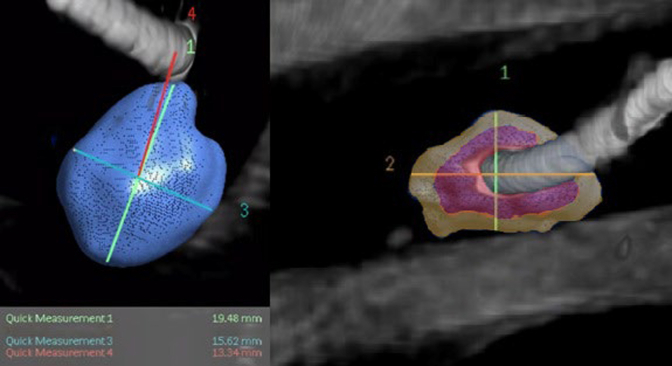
A, Quantitative assessment of tool distance from center of the target. Using this image obtained from cone beam CT scan, the center of the nodule is found (intersection between green and teal lines). A measurement is then obtained from the center of the target to the center of the catheter (red line) to determine the distance from the center; in this case, it is 13.34 mm (or 4.45× catheter diameter). The needle is not seen here because it has been deployed away from the viewer. All nodule measurements were obtained with the same method. B, Qualitative assessment of a tool in relationship to the center of a nodule. The catheter is in the center of the target. The image is divided in thirds with the pink zone representing the center, the purple zone representing the off-center or inner third, and the outer brown zone representing the at edge or outer third.
